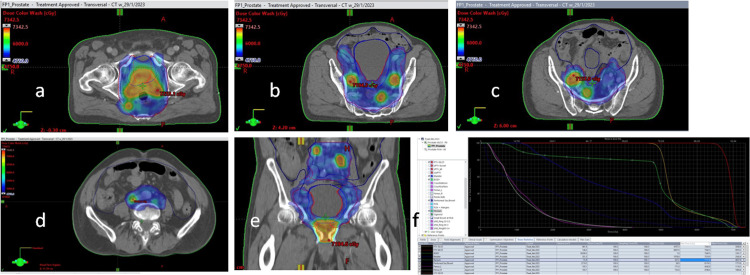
Radiotherapy treatment planning CT images showing targets and organs at risk (color lines) and radiation dose clouds (increasing dose from blue to red). (a) Axial image at the prostate gland level. (b and c) Axial images at the mid-pelvis level. (d) Axial image at the upper pelvis level. (e) Coronal plane. (f) Dose-volume histograms.
